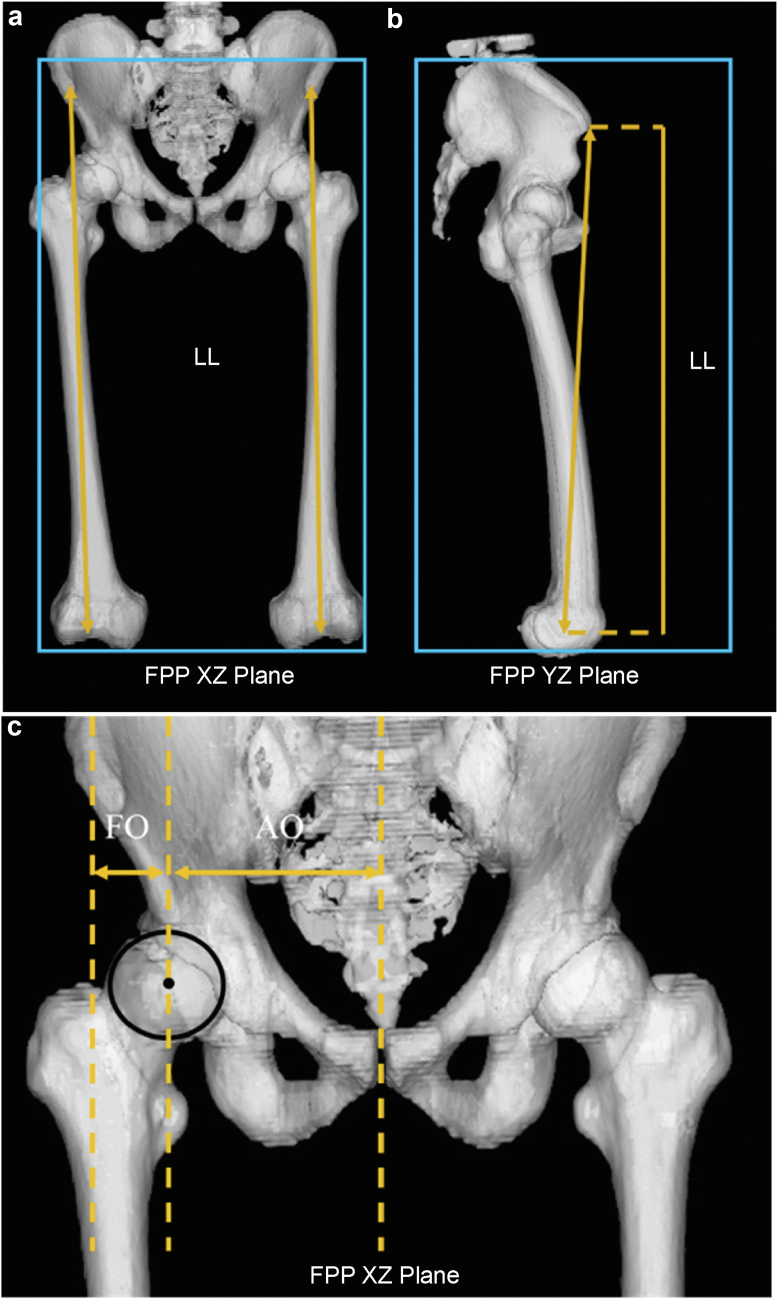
Three-dimensional computed tomography analysis. Leg length (LL), defined as the distance projected onto the frontal plane projection (FPP) from the anterior superior iliac spine to the center of the knee in the XZ plane (a) and YZ plane (b). Both the femoral offset (FO) and acetabular offset (AO) are defined as the lengths projected onto the FPP XZ plane in the neutral position (c). FO is the distance between the center of the femoral head, the center of the cup, and the axis of the femur. AO is the distance between the pubic symphysis and the center of the femoral head or cup. The global offset is the sum of FO and AO.
